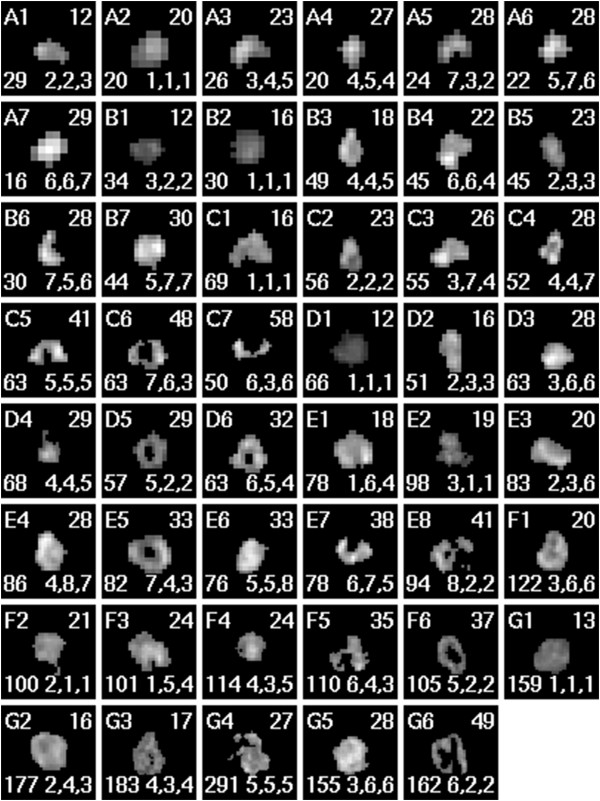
Tumors of various size, shape, mean intensity and heterogeneity are shown as assayed via FDG-PET. The letter-number at the top-left indicates the set and *ζ*-rank within that set. The number at the top-right is the rounded *ζ* value. The number at the bottom-left is the area of the tumor given in pixels. The sequence of numbers below is the ranks (within the single set) given to the image by the oncologist, the resident and the professional, respectively. The images above were rescaled via nearest-neighbor filtering to a convenient display size; thus, the sizes shown should not be compared directly.
